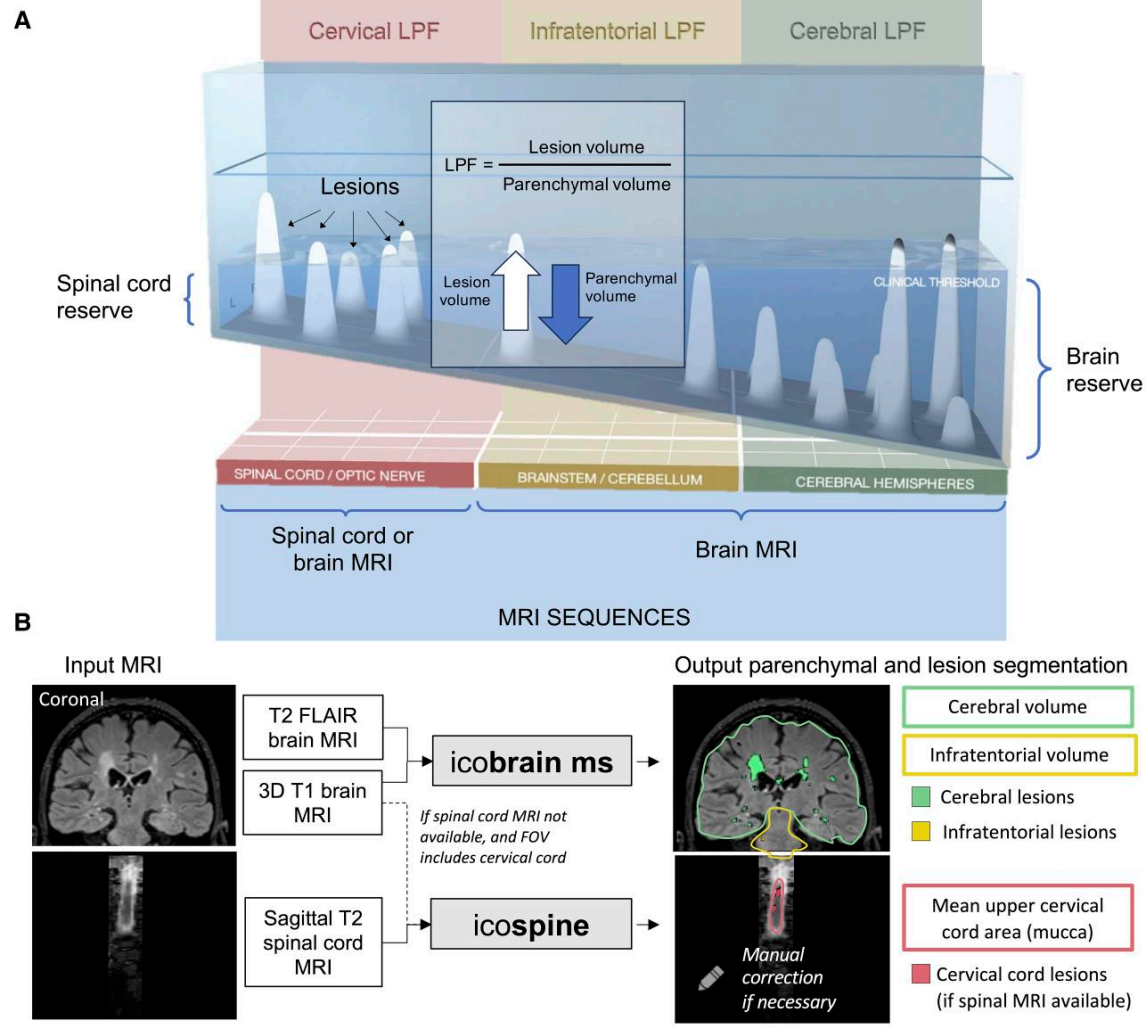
The topographical model of MS and the lesion parenchymal fraction (LPF) framework. (A) The topographical model conceptualizes the central nervous system (CNS) as a pool of reserve with increasing levels of depth, with the spinal cord at the shallow end, the brainstem and cerebellum with intermediate depth and the cerebral hemispheres comprising the deep end. Focal T2 lesions are represented as topographical peaks that rise up from the pool base. The topographical distribution drives the clinical picture for an individual patient, with functional system deficits referable to lesion localization. The single view shown represents an individual patient’s disease at a single point in time; the water is translucent, with both above-threshold clinical findings and subthreshold lesions shown. The combined volume of above-threshold topographical peaks corresponds with the degree of accumulated disability. Progression is depicted as these peaks becoming increasingly unmasked as reserve declines across CNS compartments, with brain reserve noted at right and spinal cord reserve noted at left. In the inset box, lesion parenchymal fraction (LPF) is defined as a ratio of lesion volume divided by regional parenchymal volume, serving as a quantified measure of the topographical model principles. LPF rises when lesion volume increases or as parenchymal volume declines. LPF is derived from MRI sequences using regional lesion and parenchymal volumes in the three principal topographical model compartments: cervical cord (in red), infratentorial (in yellow) and cerebral hemispheres (in green). (B) Imaging method for lesion and regional parenchymal measurement from MRI. Brain MRI input was processed by icobrain software for brain lesion and parenchymal segmentations. Spinal cord MRI and brain MRI with cervical cord were processed by icospine software for cervical cord lesion and parenchymal segmentations. Visual representation of segmented outputs shows parenchymal volume outlines, and individual lesions, colour-coded according to the three topographical model regional compartments.
